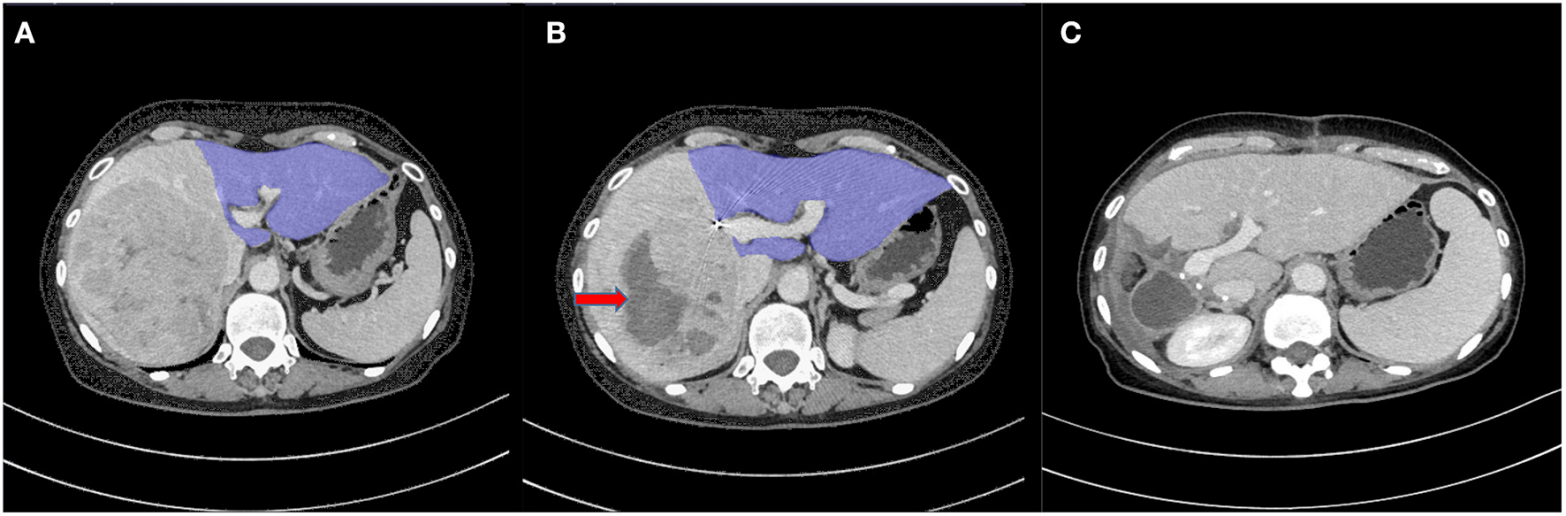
(A) Contrast-enhanced CT image before sequential portal vein embolization (PVE) + radiofrequency ablation (RFA) (PVE+RFA) shows a 106 mm × 103 mm tumor in the right lobe. The future liver remnant (FLR) (the blue part) was 385 ml (32%). (B) Eight days after PVE+RFA, the FLR had increased to 508 ml (42%) (the blue part). Red arrow shows tumor necrosis induced by RFA. (C) CT image at follow-up 48 days after liver resection shows a continued FLR growth.
